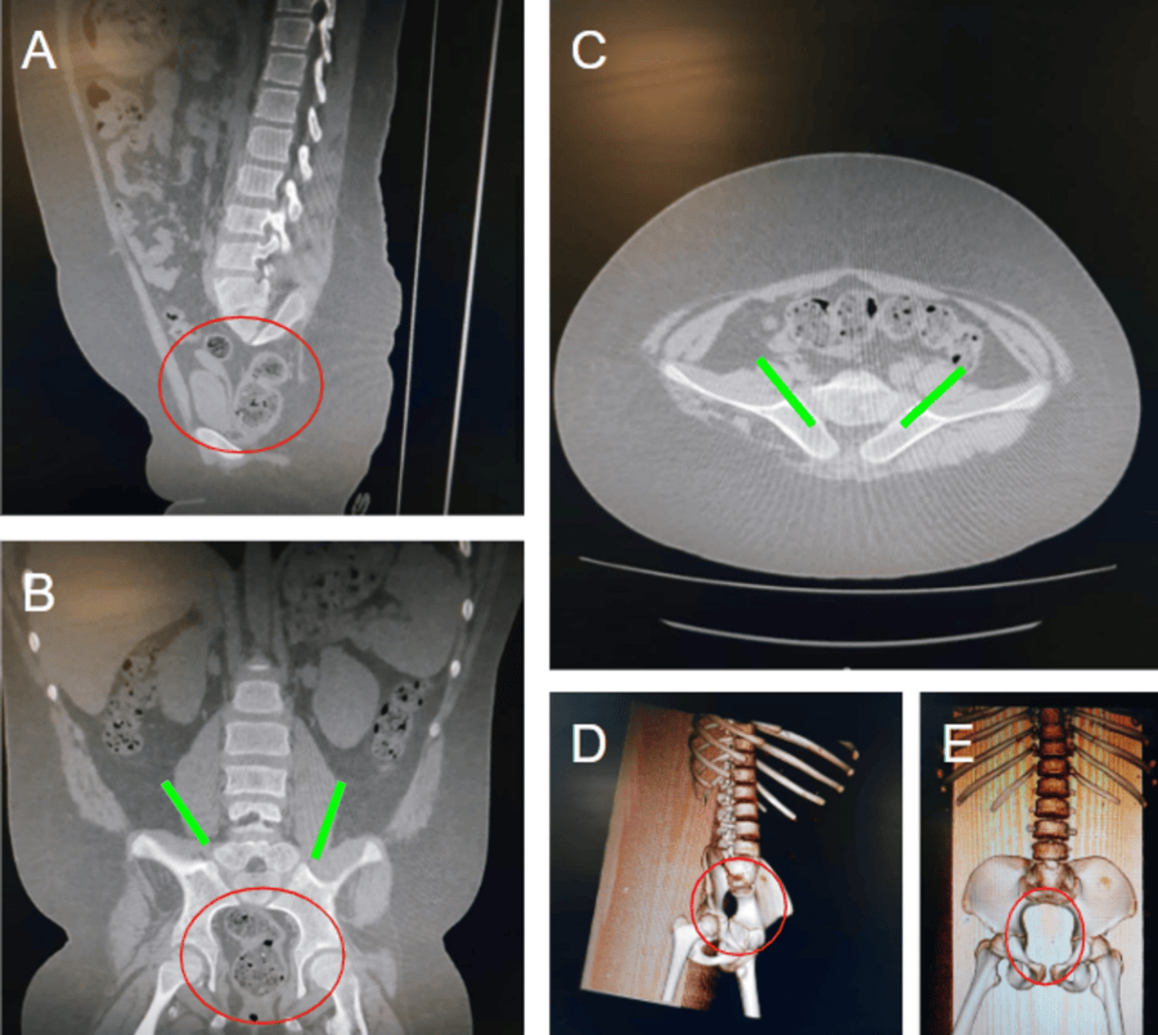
(A) Sagittal, (B) coronal, (C) axial, and (D,E) 3D reconstructed CT images showing bilateral iliac bones articulating with the S1 vertebra with reduced distance between them. There is an absence of the rest of the sacral vertebrae and coccyx. Green lines: Bilateral iliac bones articulating with the S1 vertebra, with reduced distance between them. Red circles: Absence of the rest of the sacral vertebrae and coccyx.
